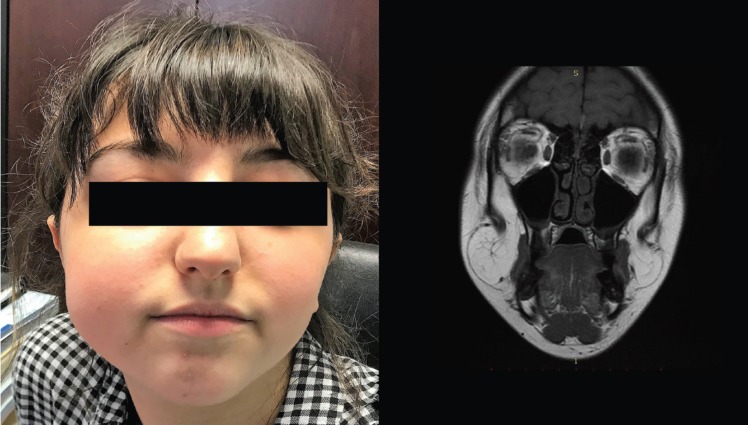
A) Preoperative photograph showing the asymmetry of the face. B) MRI imaging of the bilateral masses.
